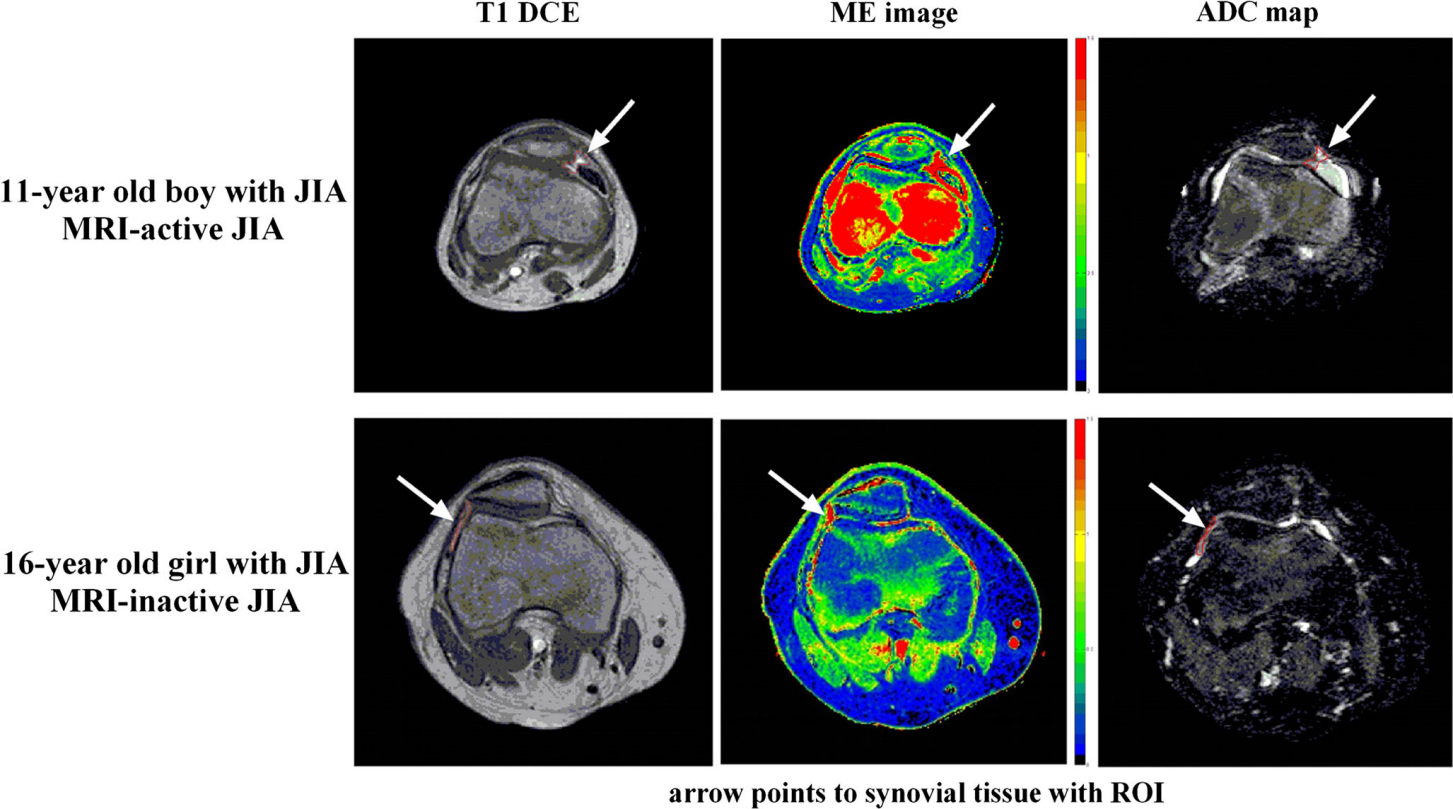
Example of a T1 dynamic contrast-enhanced image and parametric maps (maximum enhancement (ME) and apparent diffusion coefficient (ADC)) of an MRI-active JIA patient (*upper row*) and MRI-inactive JIA patient (*lower row*) with demonstration of region of interest (ROI) placement. Arrows point to the ROIs. ROIs were placed in synovial tissue. Joint effusion (if present) was not included in the ROI, as can be seen in the T1 DCE image of the 11-year old boy with MRI-active JIA
